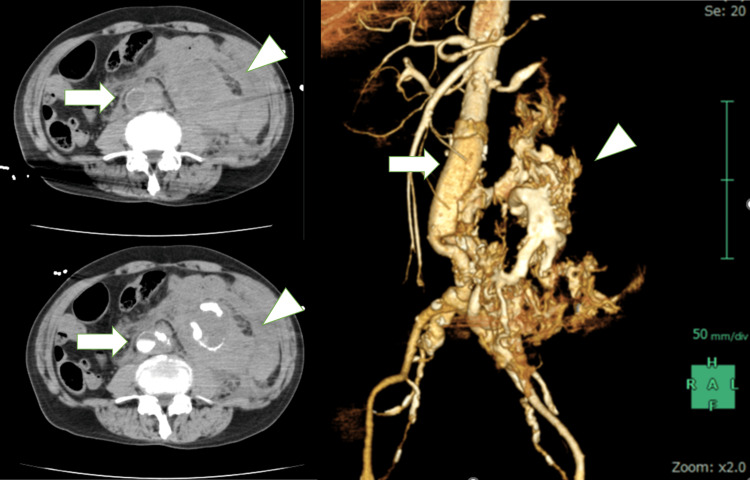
Emergency contrast-enhanced computed tomography A contrast-enhanced computed tomography scan revealed massive retroperitoneal hemorrhage (arrowheads) around the bifurcated graft (arrows). There was no residual or recurrent aneurysm. It was difficult to identify the bleeding site.
